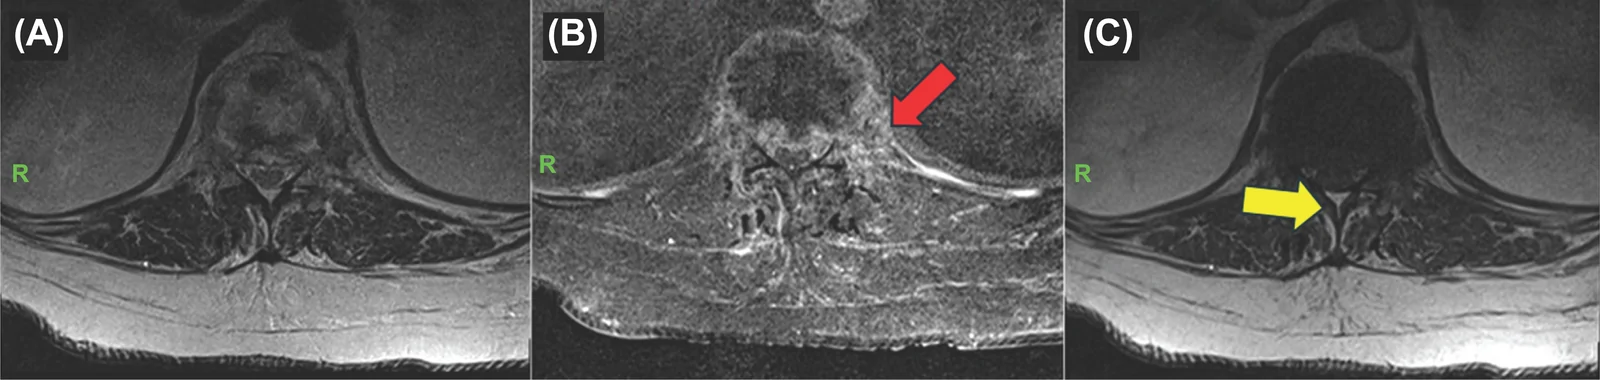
Thoracolumbar MRI at T12, axial T1-weighted (A), post-gadolinium T1-weighted (B), T2-weighted (C). Hypointense marrow signal in T12 (A, C) with intense post-contrast enhancement (B). Subligamentous collection (red arrow) contributes to ≈60% spinal stenosis and compressive myelopathy (yellow arrow). Abbreviation: MRI, magnetic resonance imaging.
